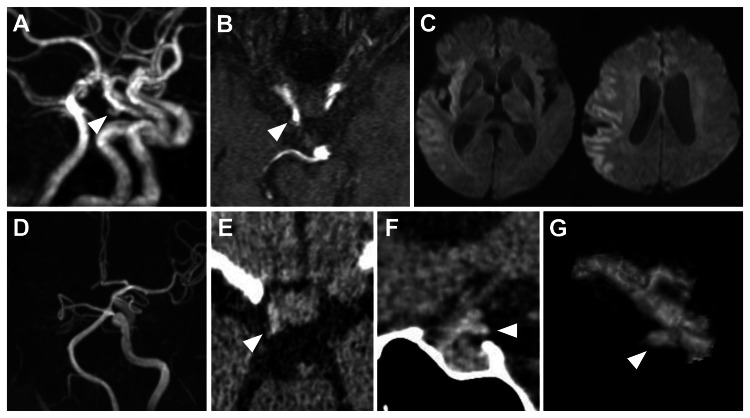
Preoperative images Three-dimensional (A) and axial (B) magnetic resonance angiography (MRA) performed 4 years ago showing a right internal carotid artery–posterior communicating artery (IC-PC) aneurysm (arrowhead). (C) Preoperative diffusion-weighted imaging (DWI) showing faint hyperintense areas in the right middle cerebral artery territory (DWI-Alberta Stroke Program Early Computed Tomography [CT] Score, 4 points). (D) Preoperative MRA showing right internal carotid artery occlusion. Preoperative plain axial (E) and sagittal (F) CT showing hyperdensity in the occluded vessel and the right IC-PC aneurysm area (arrowhead). (G) Reconstructed image showing hyperattenuated areas of hyperdensity on preoperative plain CT showing right IC-PC aneurysms (arrowhead) within the occluded internal carotid and middle cerebral arteries
